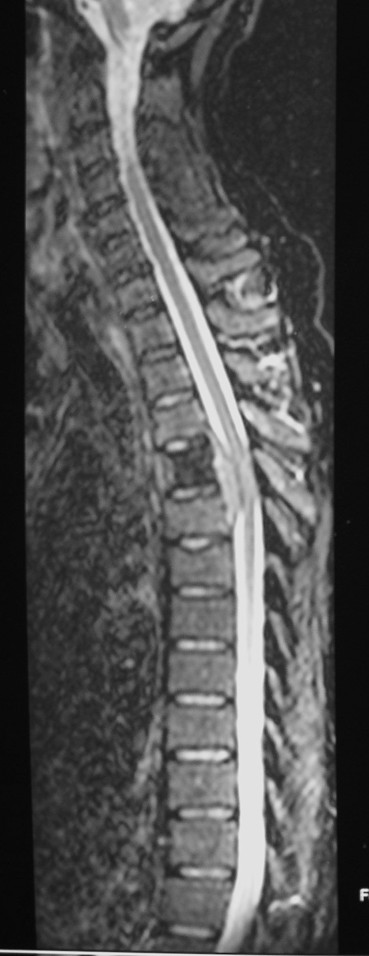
T2W MRI of Thoracic spine.
